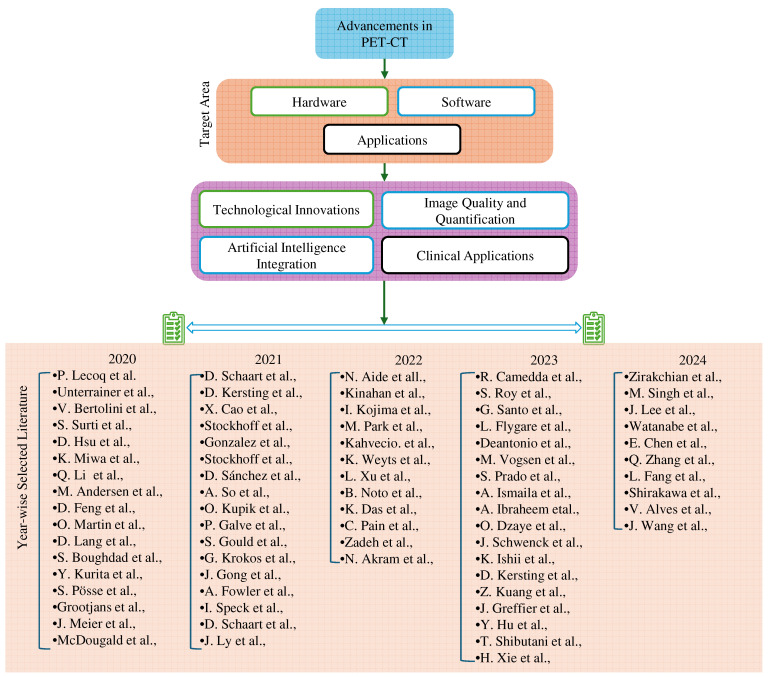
Selected articles with top achievements over the past 5 years focused on PET-CT and multi-model medical imaging modality [5,6,7,8,9,10,11,15,16,17,18,19,20,21,22,23,24,25,26,27,28,29,30,31,32,33,34,35,36,37,38,39,40,41,42,43,44,45,46,47,48,49,50,51,52,53,54,55,56,57,58,59,60,61,62,63,64,65,66,67,68,69,70,71,72,73,74,75,76,77,78,79,80].
